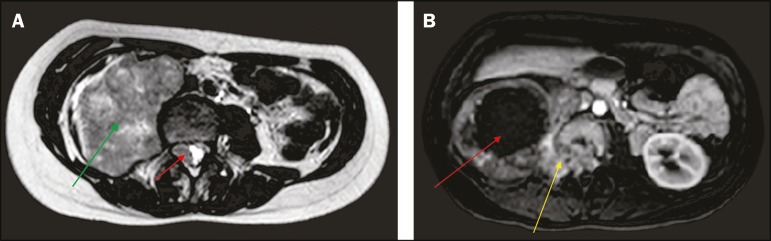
A: Axial T2-weighted MRI scan showing a huge heterogeneous
